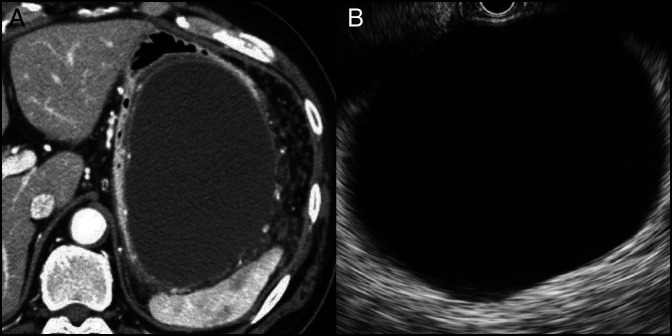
Large pancreatic pseudocyst with severe gastric compression on (A) dynamic computed tomography and (B) endoscopic ultrasound.
